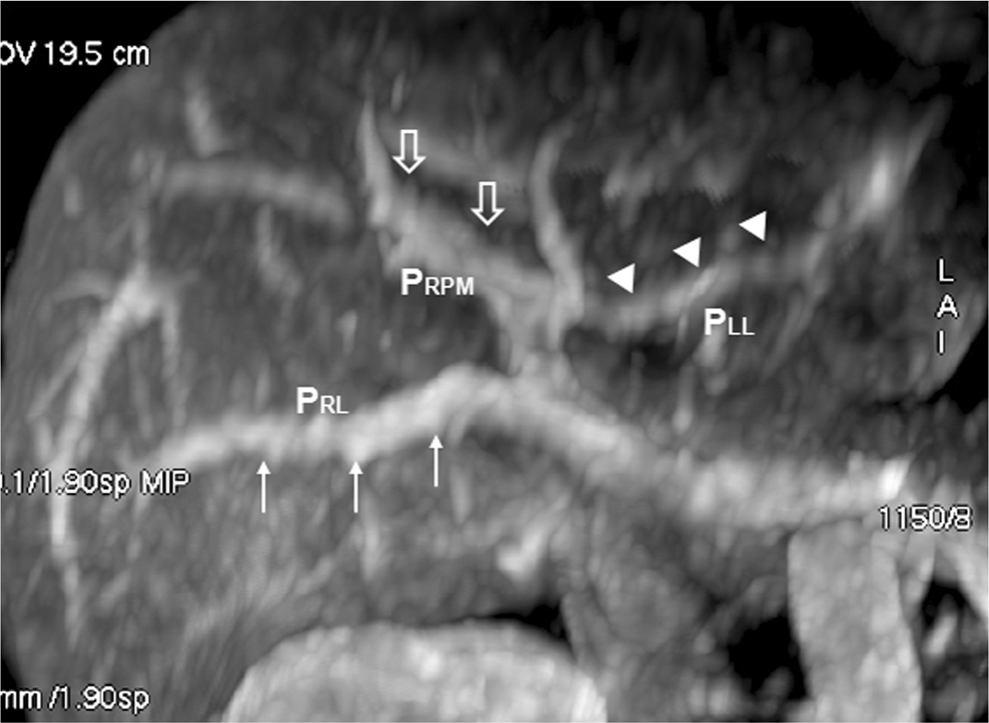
Contrast-enhanced T1 fat-sat magnetic resonance imaging (MRI) with maximum intensity projection (MIP) reconstruction revealing portal vein ramification of a Shindoh’s independent right lateral type
